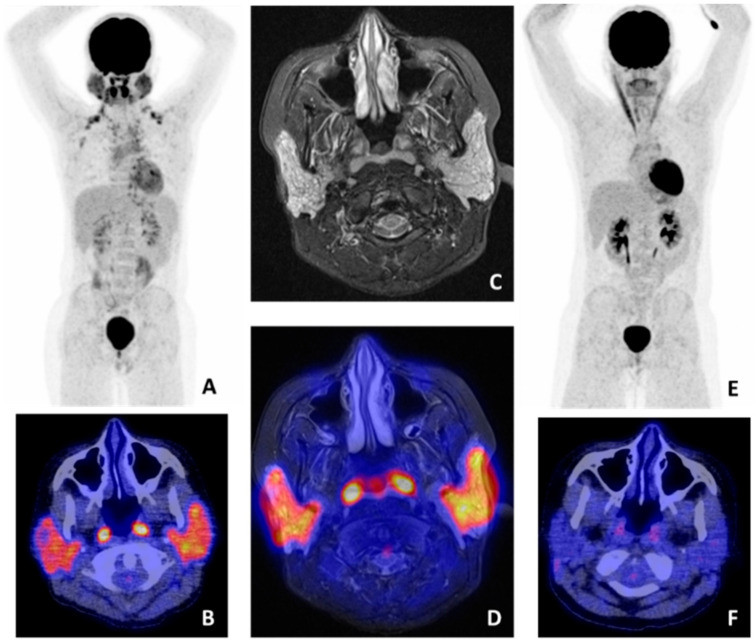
^18^F-fluorodeoxyglucose (FDG) positron emmison tomography/computed tomography (PET/CT) and magnetic resonance imaging (MRI) findings in a pSS patient with salivary gland mucosa associated lymphoid tissue (MALT) lymphoma. (A) Whole-body FDG-PET showing high heterogeneous FDG uptake in both parotid and submandibular glands. No other pathological lesions were found (axillary and clavicular regions with increased uptake represent brown fat). (B) FDG-PET/CT image showing pathological uptake in the parotid glands and physiological uptake in the tonsils. (C) MRI stir sequence showing a pathological, heterogeneous aspect of both parotid glands. (D) Manually fused FDG-PET/MRI image, showing pathological uptake in the parotid glands and physiological uptake in the tonsils. (E) Whole-body FDG-PET and (F) FDG-PET/CT image after treatment, showing no pathological uptake in the parotid glands, indicating complete remission.
